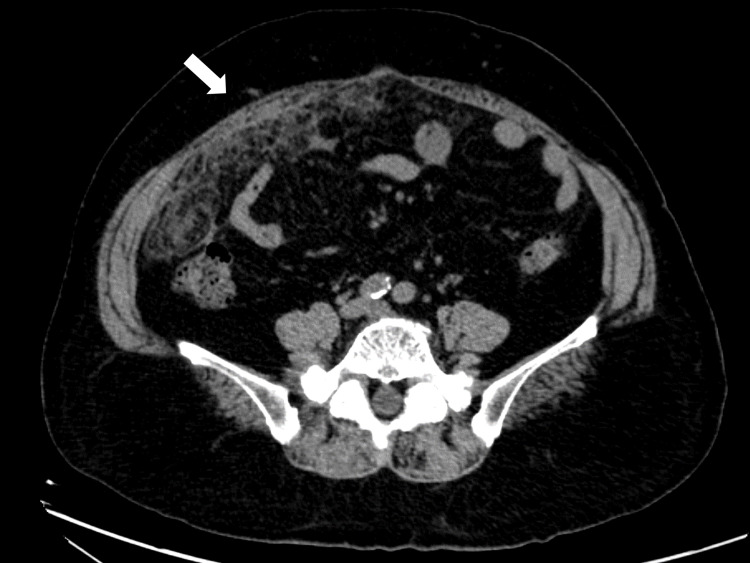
Initial abdominal CT. The image shows diffuse enhancement on the right lower peritoneum. CT: computed tomography
